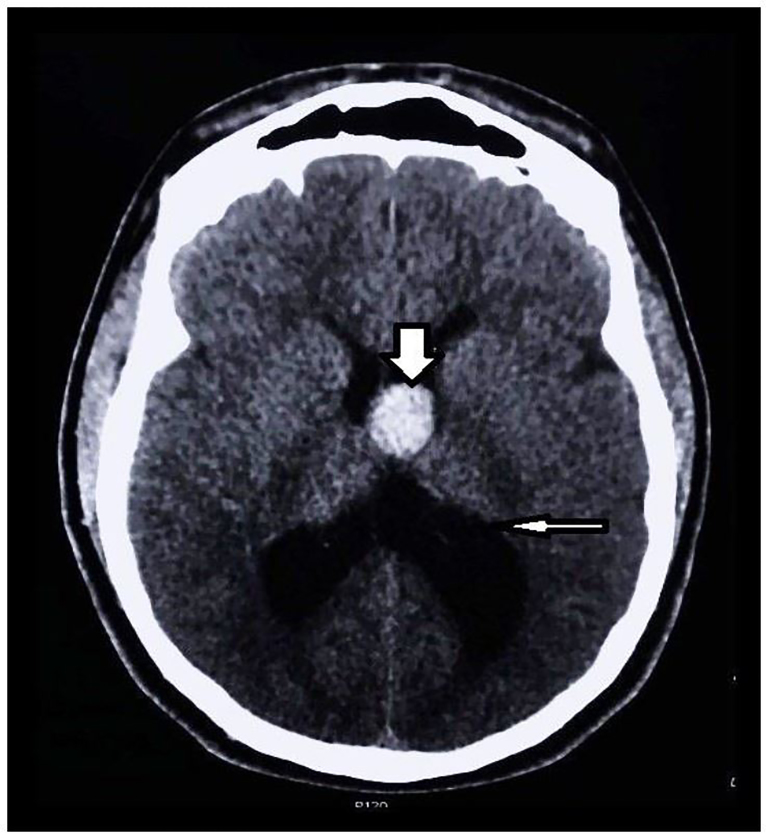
Initial noncontrast computed tomography head demonstrating the presence of the colloid cyst (thick arrow) with associated hydrocephalus (thin arrow).
